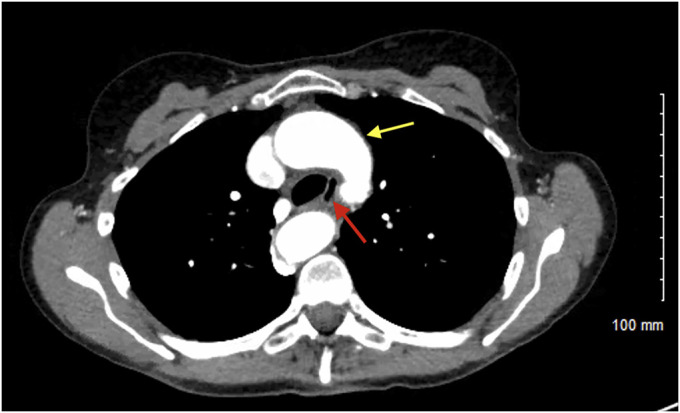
Chest computed tomography angiography demonstrating vascular ring from a double aortic arch (yellow arrow) encasing the trachea and esophagus (red arrow).
